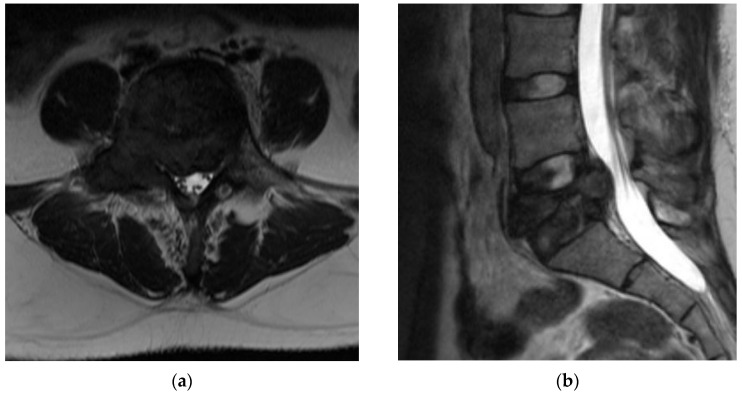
(a) Axial T2 weighted MRI cuts of L5 vertebral body show low signal intensity of the tumor body with extension into bilateral pedicles. There is mild compression of the spinal canal. (b) Sagittal T2 weighted MRI cuts of L5 vertebral body again show low signal intensity. There is retropulsion of the compressed L5 vertebral body causing narrowing of the canal.
